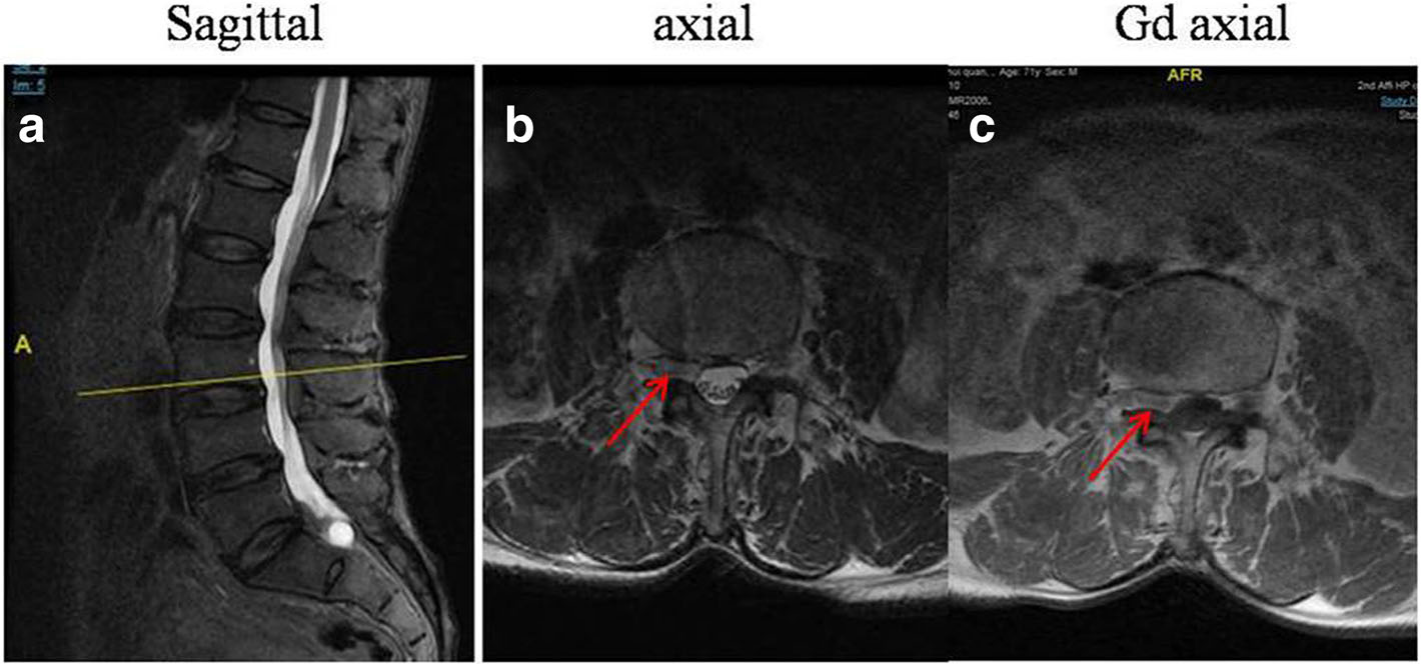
Preoperative MRI of the lumbar spine without intravenous contrast and with gadolinium- enhanced MRI. T2-weighted MRI in the sagittal plane was used to aid in localization (a). T2-weighted images in the axial planes revealed a dumbbell mass in the lateral recess (b). Gadolinium-enhanced MRI demonstrated a heterogeneous ring-like enhancement at the periphery of the mass (c)
